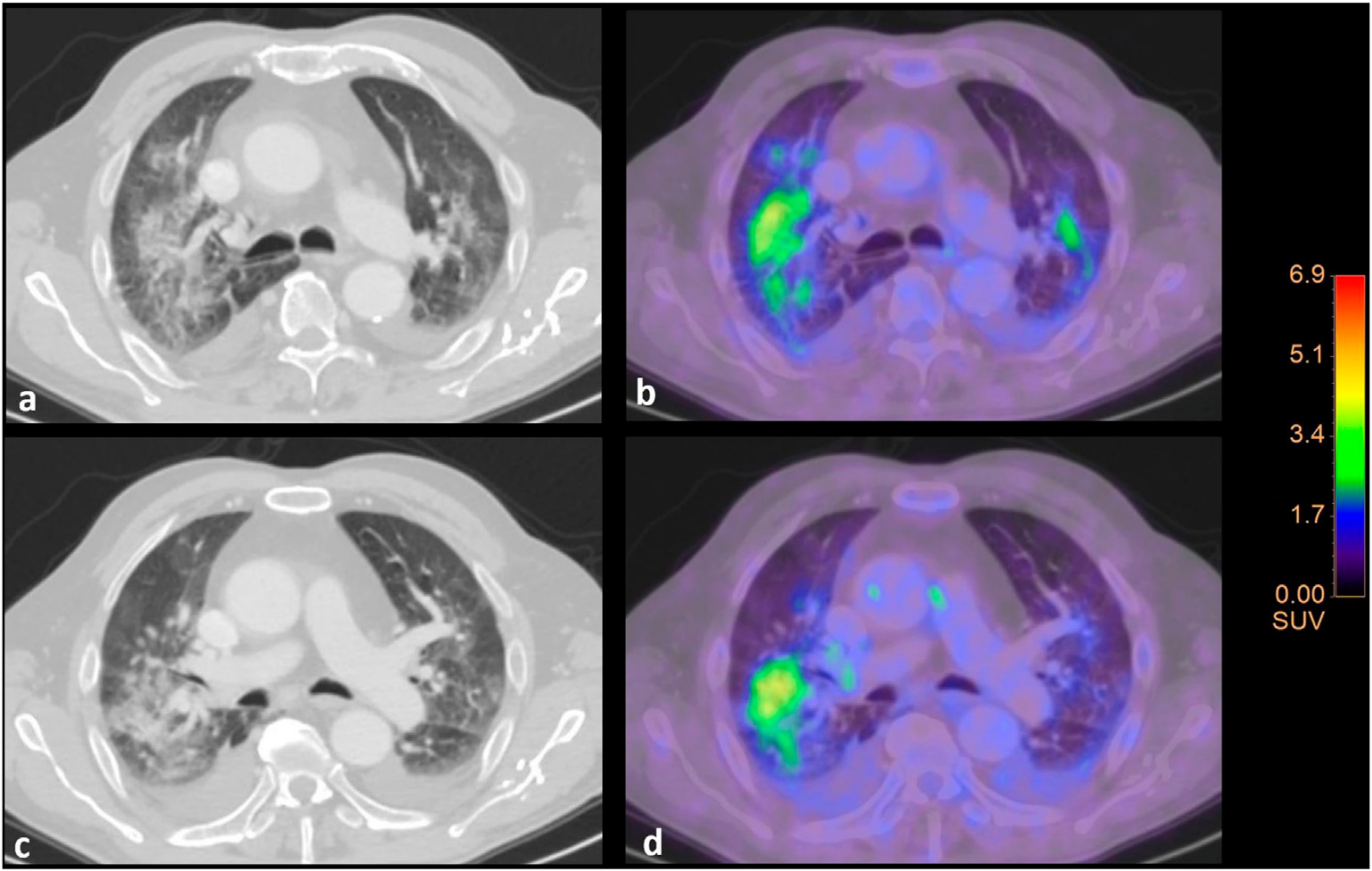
2-[^18^F]FDG PET/CT in patient of Figure 1 demonstrating diffused bilateral lung abnormalities (CO-RADS 5) (a and c) with increased inhomogeneous 2-[^18^F]FDG activity (SUV max 5.1) (b and d) as outcome imaging findings of interstitial pneumoniae by prior COVID-19 infection; bilateral pleural effusion was also present.
